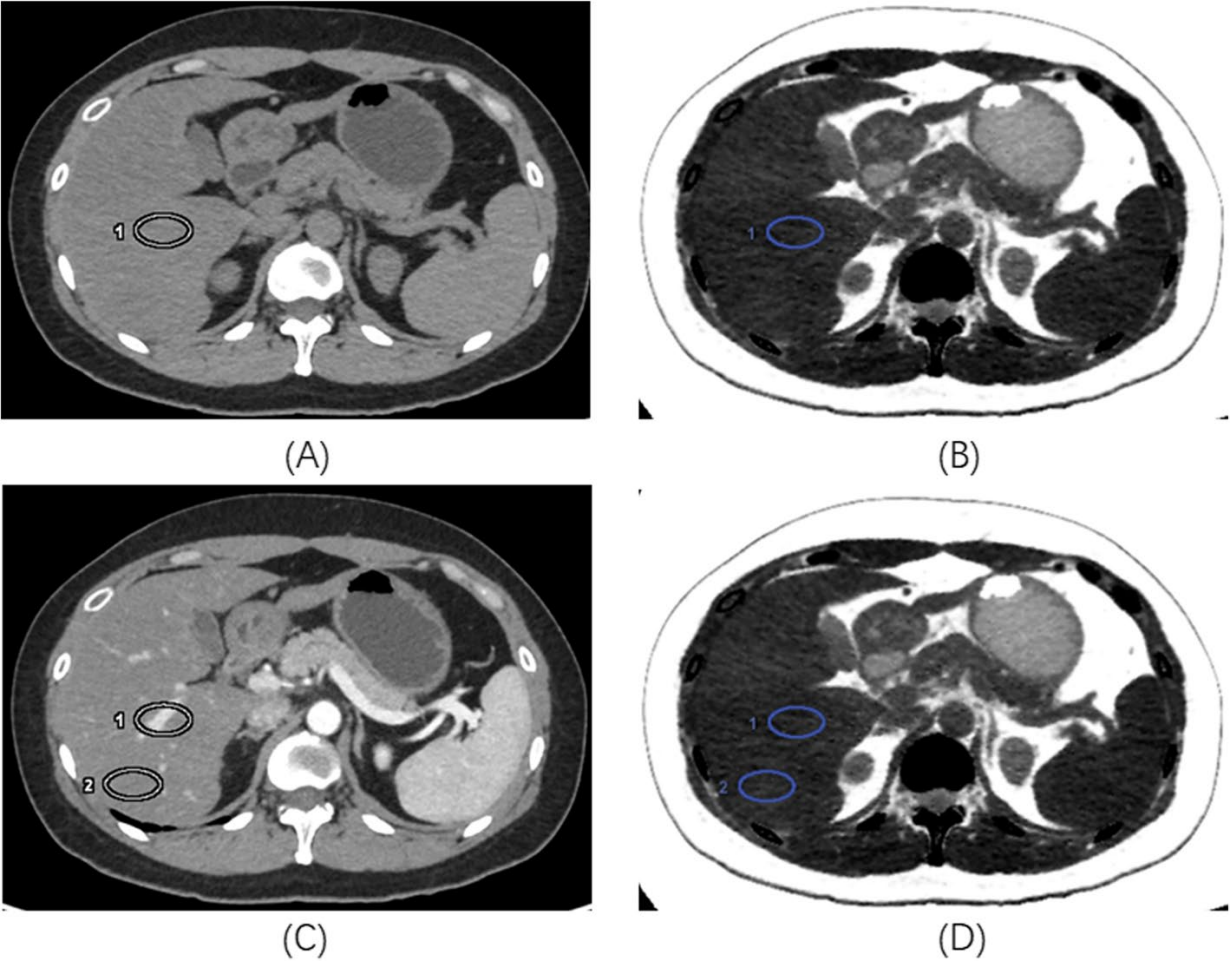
The CT images from a young man with NAFLD. A 400 mm.^2^ ROI (ROI1) was placed on the RPL on 70 keV NCP image (A) without the reference of enhanced CT images, and the FVF value was measured as 15.51% on the corresponding fat image (B). Another ROI (ROI2) was carefully placed avoiding the blood vessel according to the enhanced 70 keV PVP images (C), and the FVF value was measured as 16.94% on the corresponding fat image (D). Note: *FVF* fat volume fraction, *NAFLD* nonalcoholic fatty liver disease, *NCP* non–contrast enhanced phase, *RPL* right posterior lobe
